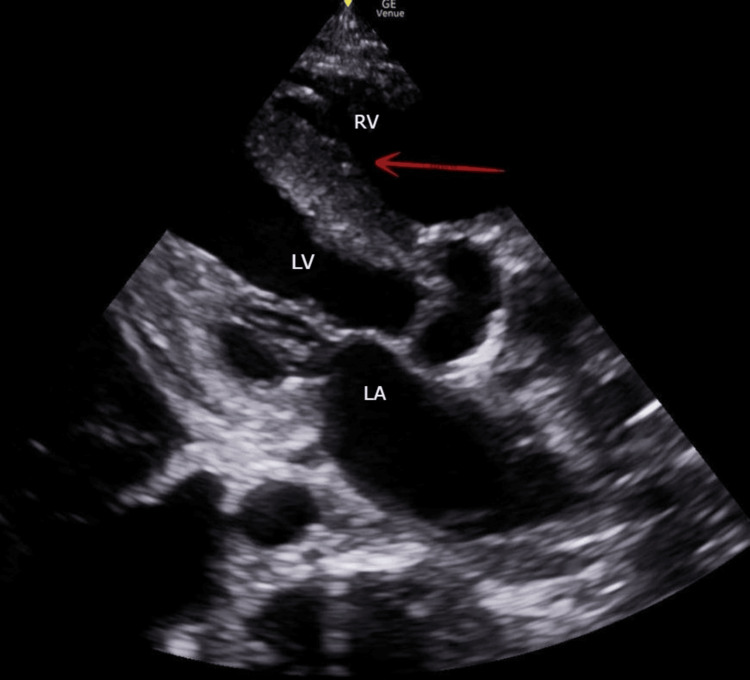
Deviation of the interventricular septum on a parasternal long-axis view (red arrow), and the heart chambers are labeled as RV, LV, and LA RV: right ventricle; LV: left ventricle; LA: left atrium
